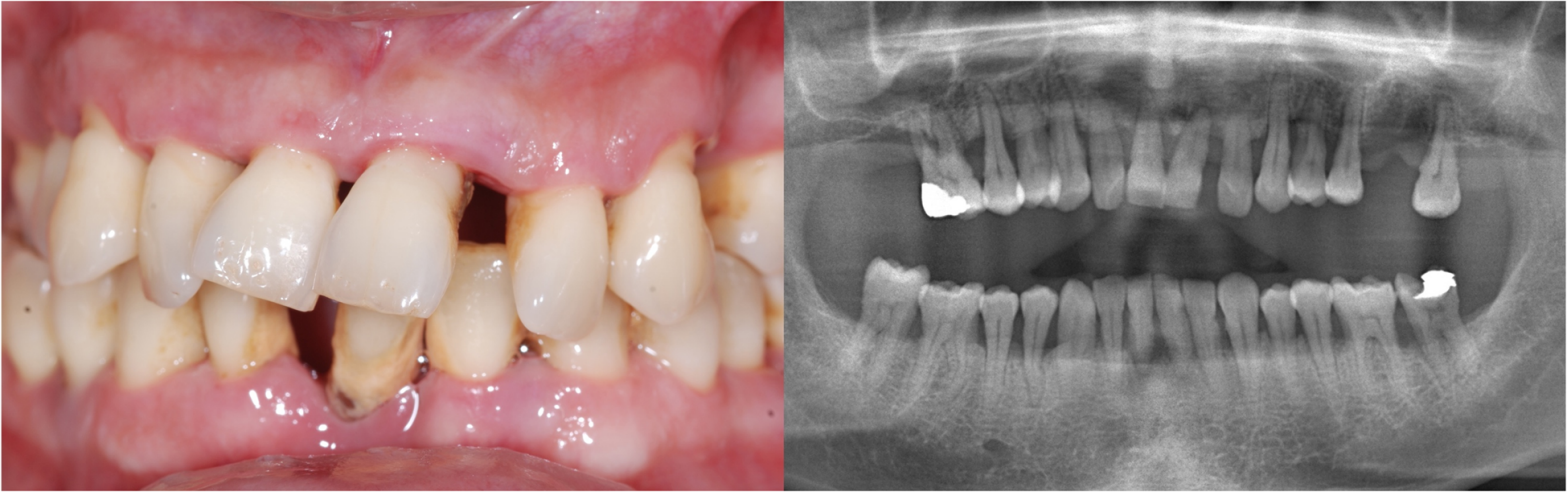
Clinical images of 42-year-old male patient receiving a full-arch maxillary reconstruction. Preoperative intraoral picture (right) and orthopantomography (left) showed the need for full-arch prosthetic reconstruction of the maxilla
